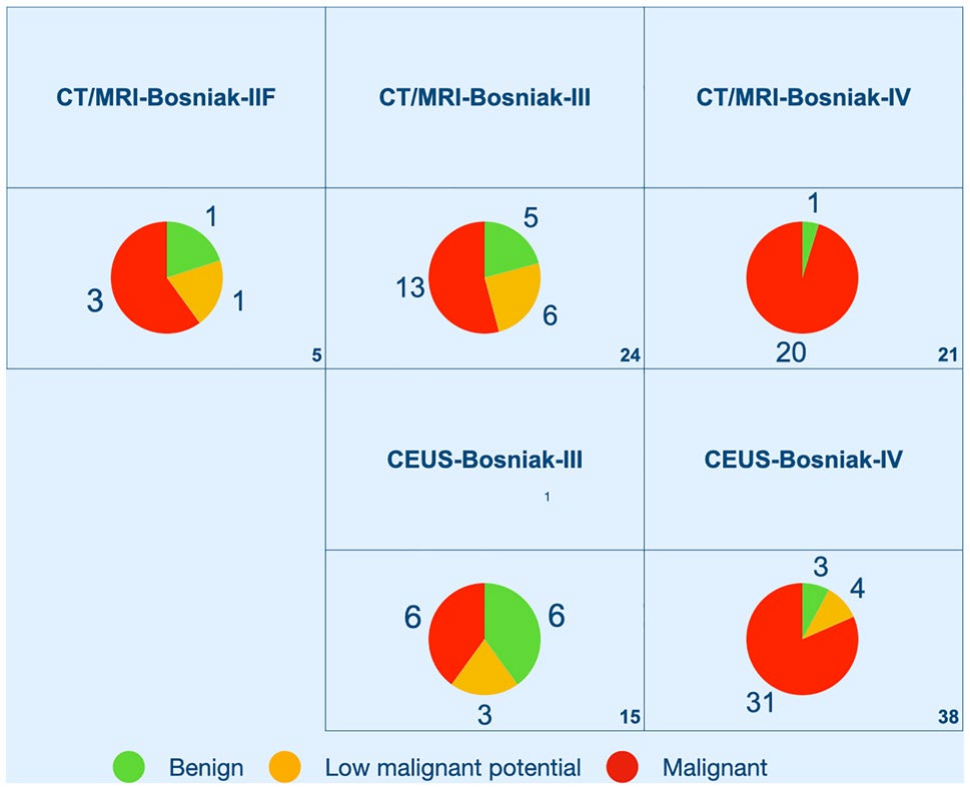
Comparison of CEUS-Bosniak classification and CT/MRI-Bosniak classification in resected cysts. Pie charts show the percentage of patients with benign (green), low malignant (yellow), and malignant findings (red); numbers (bottom right) indicate the number of patients
